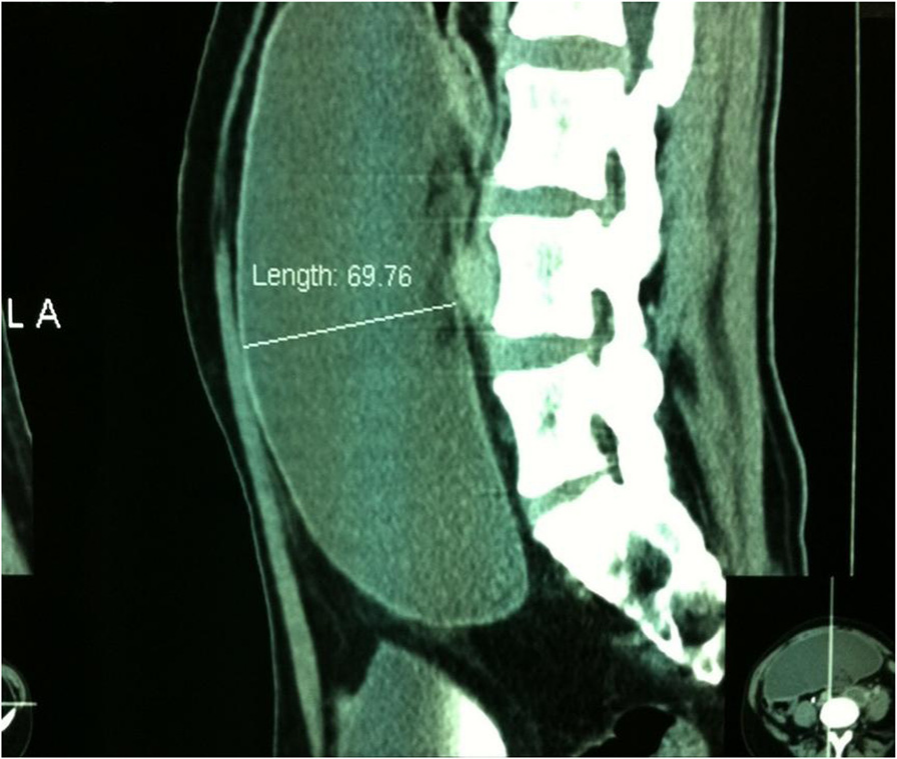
Sagital Angio-CT showing size of spleen.
